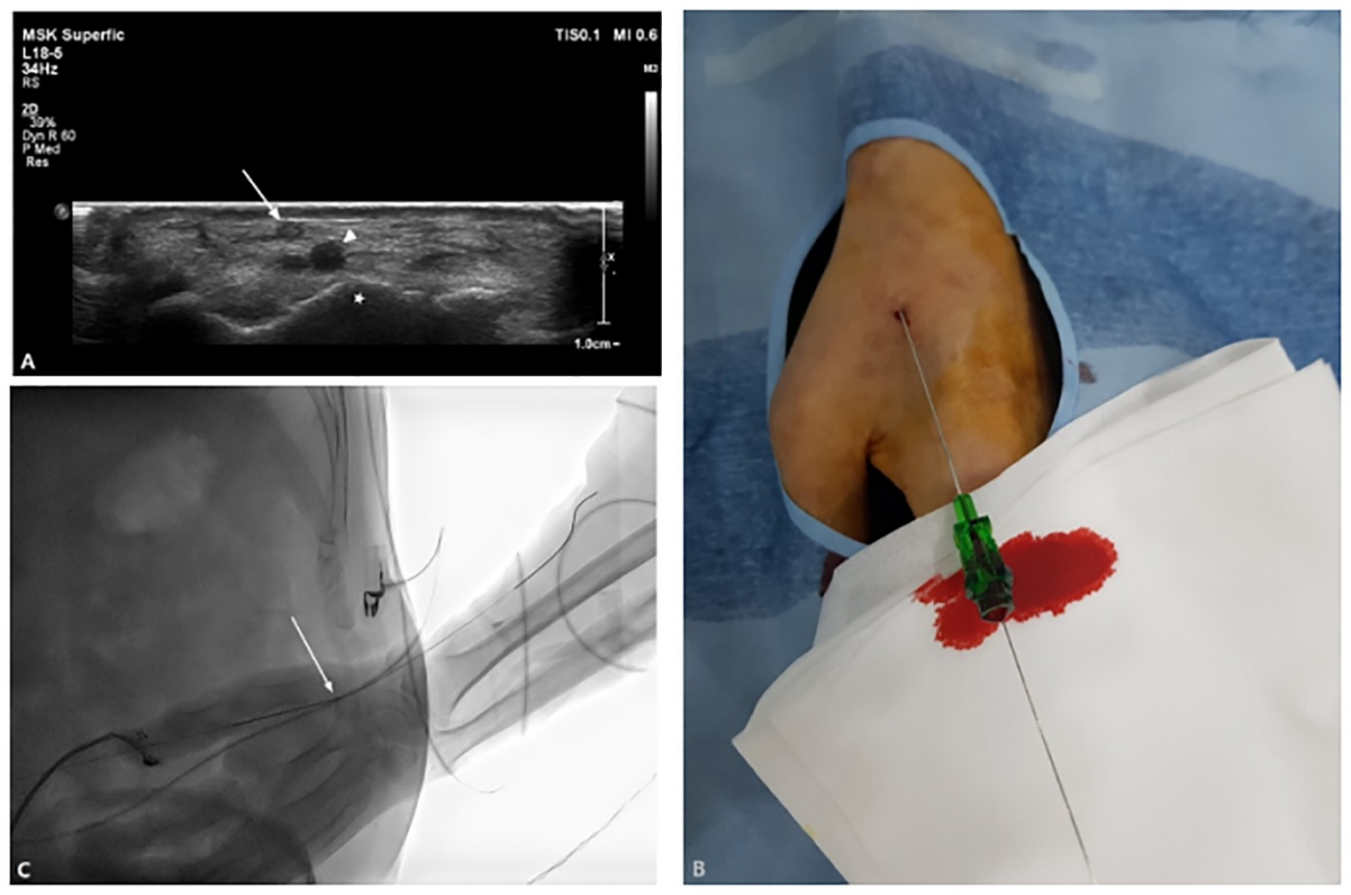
(a) The ultrasound image of the anatomical snuffbox shows the superficial branch of the radial nerve (arrow), the distal radial artery (arrowhead), and the trapezium bone (asterisk). (b) The distal radial artery at the snuffbox was punctured using a microneedle. (c) The fluoroscopic image shows the punctured distal radial artery at the snuffbox (arrow) and the guidewire passed through the proximal radial artery.
